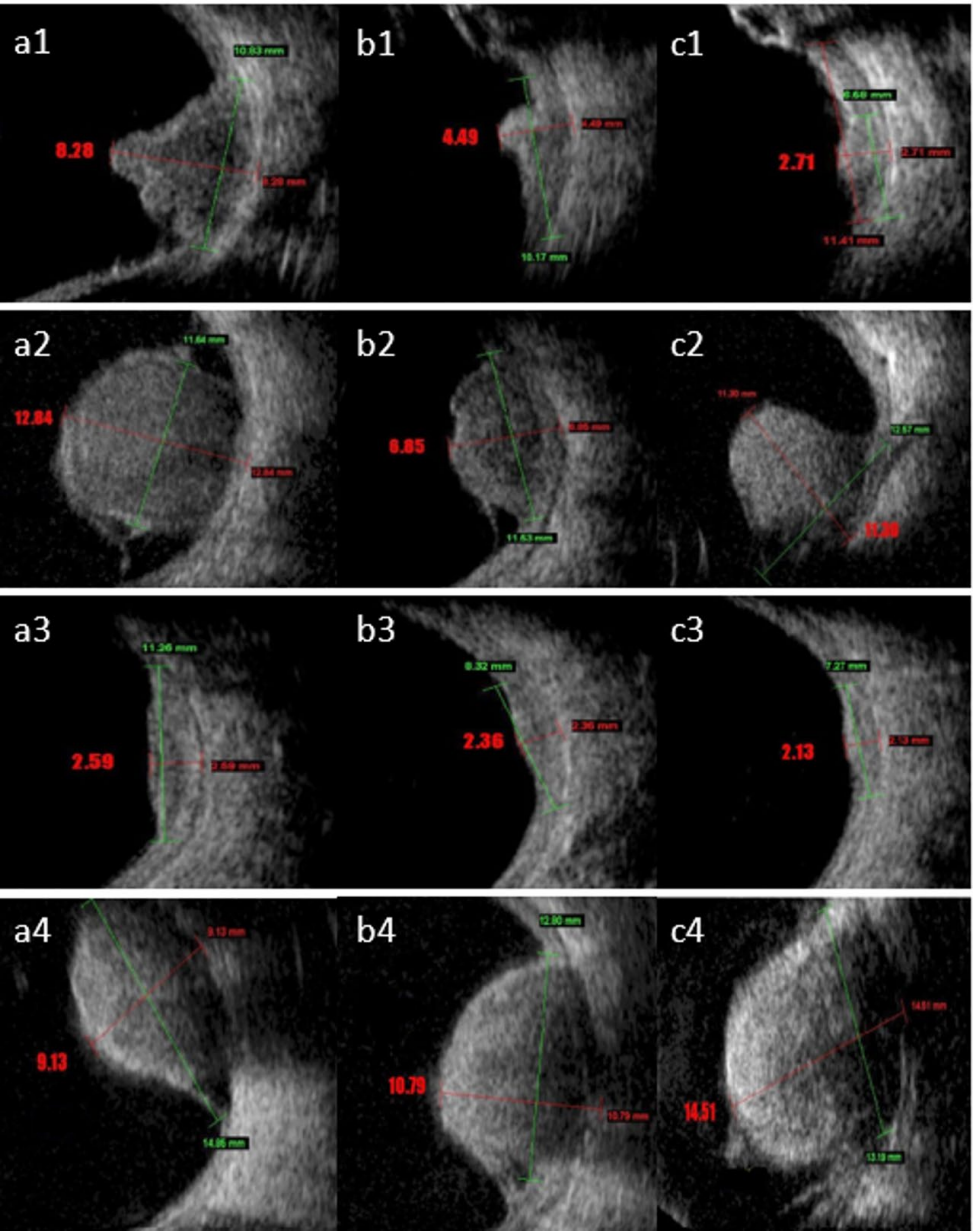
B-Mode ultrasound images of four different patients with Decrease, Other, Stop, and Increase response pattern at the time of diagnosis (a1, a2, a3, and a4), one year (b1, b2, b3, and b4), and two years (c1, c2, c3, and c4) after treatment with Ru-106 plaque brachytherapy.
